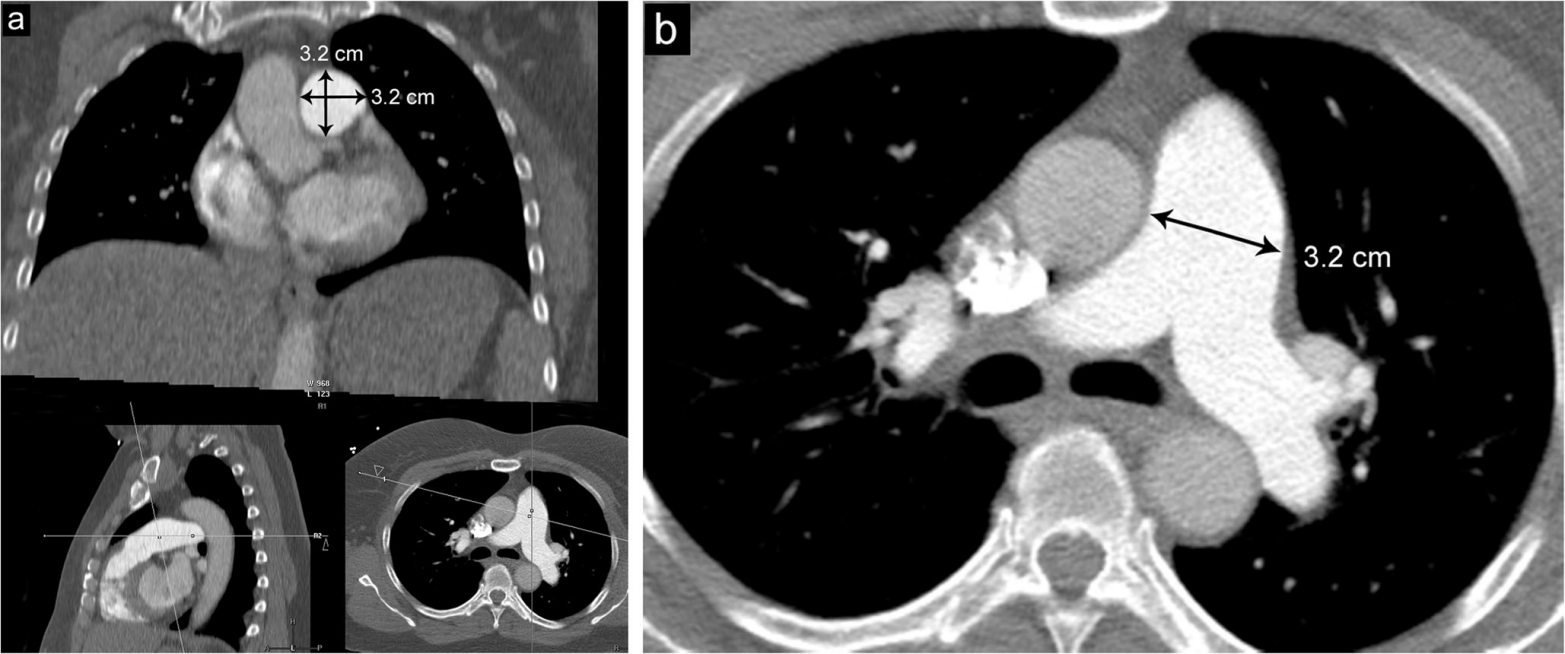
Pulmonary artery measurement. a CT imaging demonstrating double oblique measurement technique through the main pulmonary artery. b Axial CT image demonstrating transverse measurement through the main pulmonary artery at the level of the right pulmonary artery bifurcation
